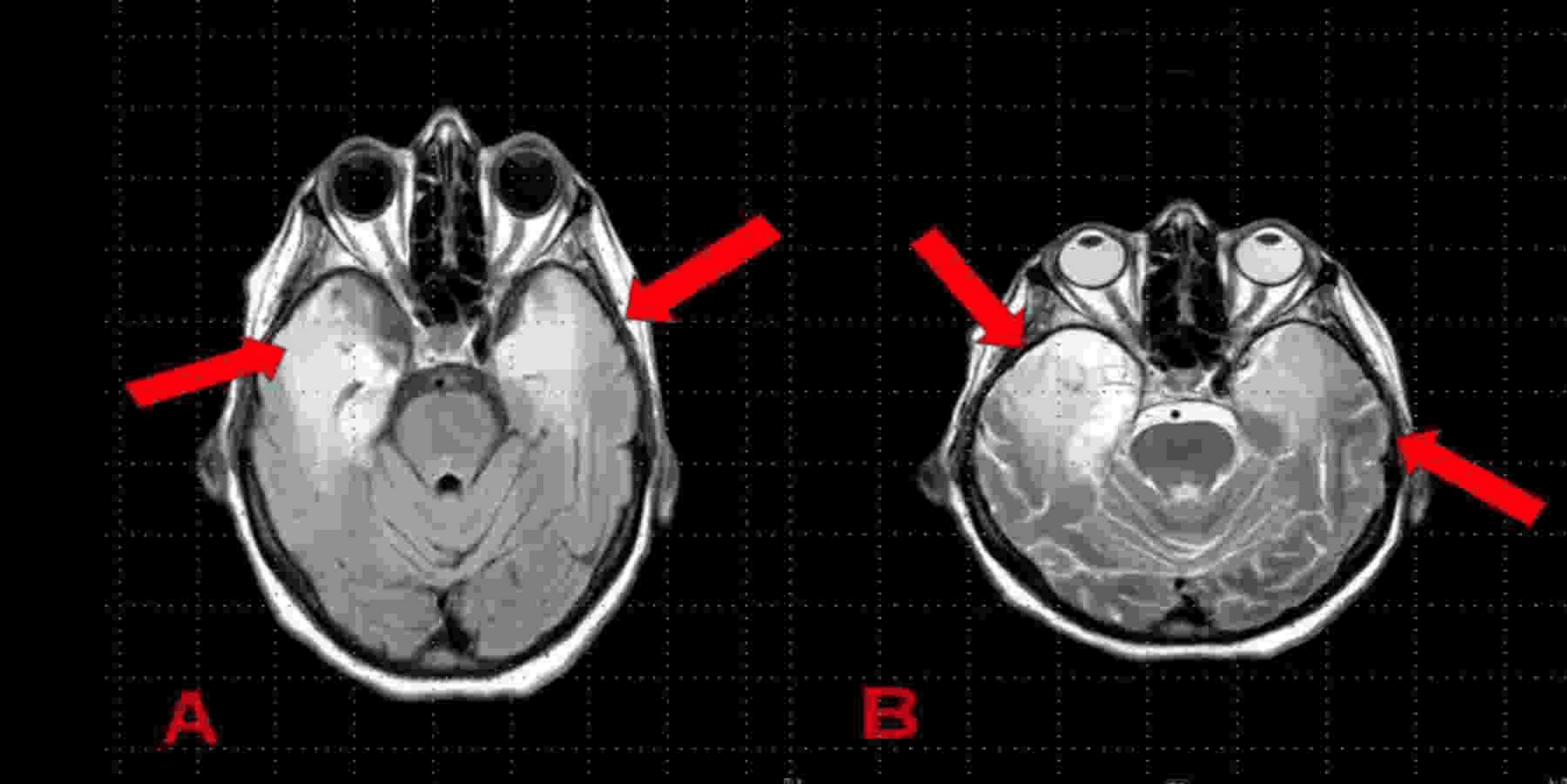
Brain MRI showing diffuse abnormal T2/fluid-attenuated inversion recovery hyperintense signal in the bilateral temporal lobes (A) and bilateral hippocampi (A, B) (red arrows). The MRI was taken on day six after the patient presented with worsening symptoms of confusion and a Glasgow Coma Scale score <5.
